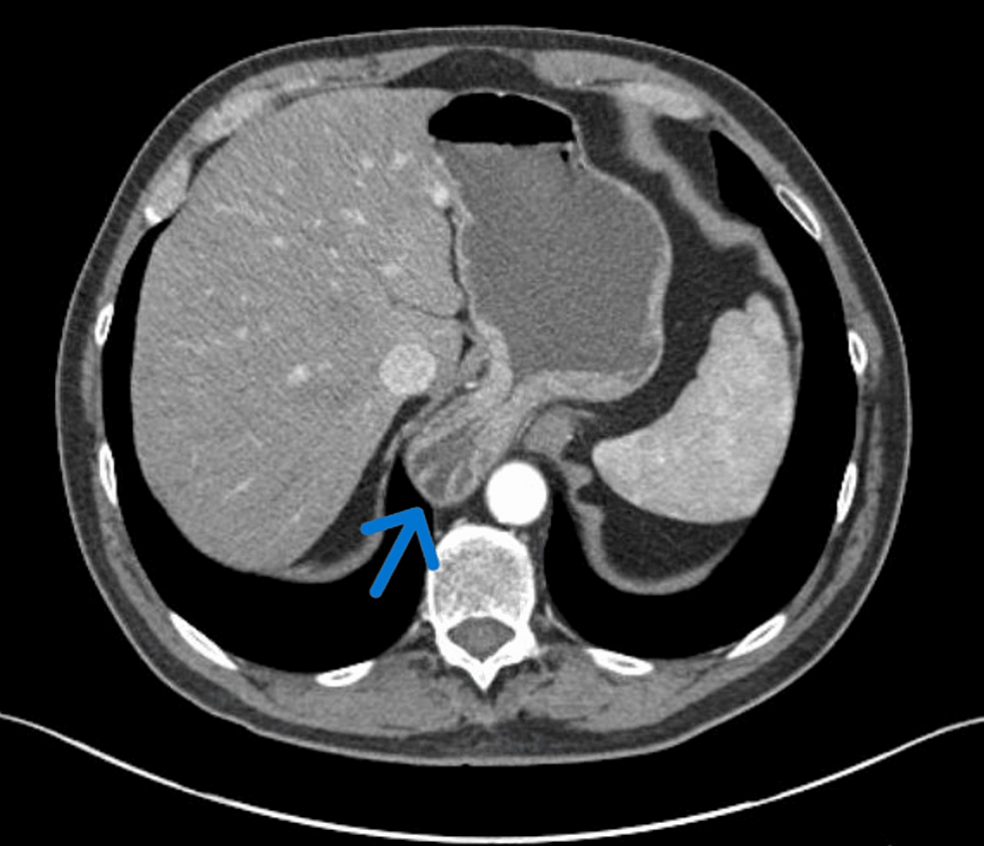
CT enterography showing a hiatal hernia without any evidence of a gastric mass.
